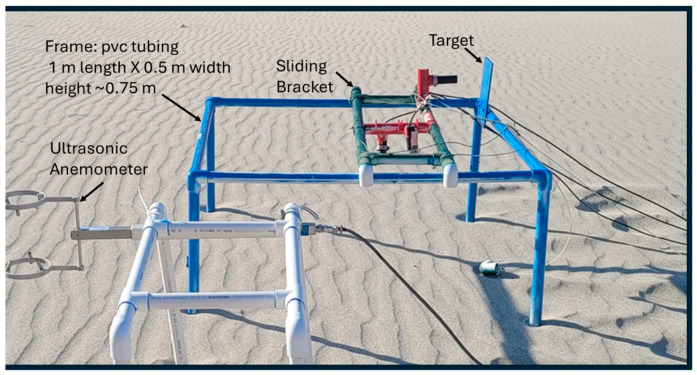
The ripple rack mount and the ToughSonic^®^ bracket, paired with a 3D, ultrasonic anemometer (SATI/3A-130201). The cables lead to the power supply, data acquisition, and recording hardware.
